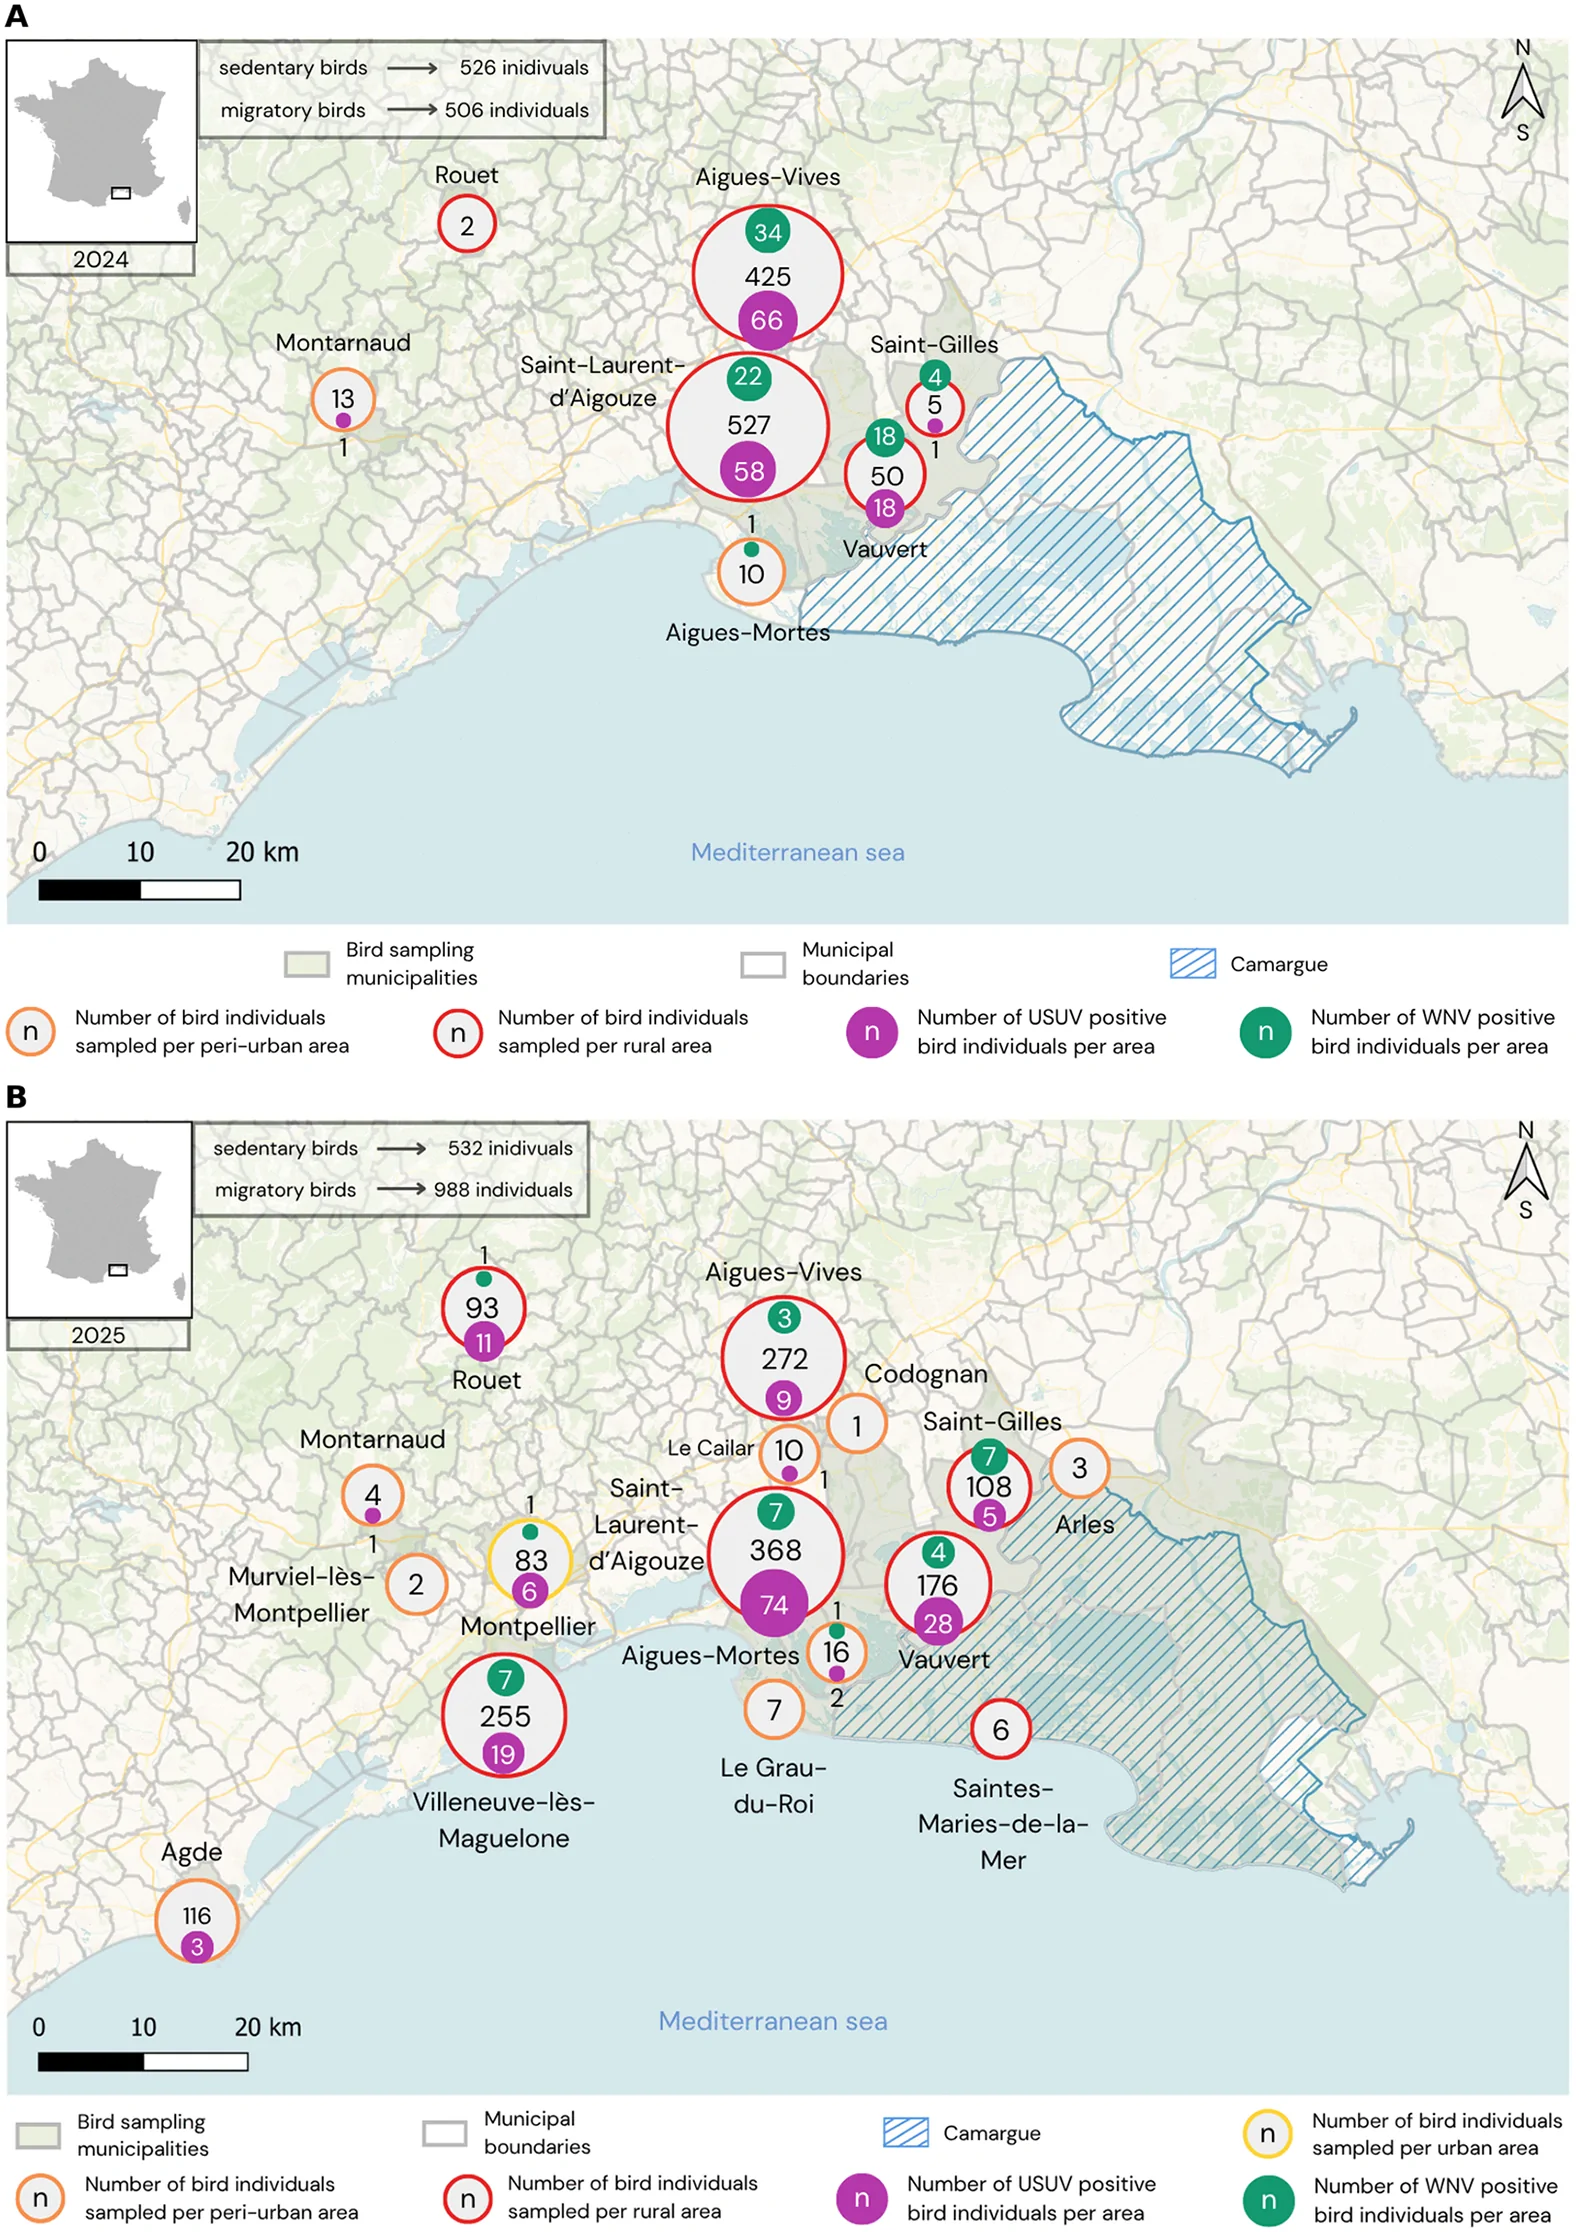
Spatial distribution of USUV and WNV infections in birds captured in 2024 (A) and 2025 (B). Each circle is centred on a sampling municipality; its size and inset number indicate the total number of individual birds captured there, and its outline colour indicates the locality type as defined in the Methods (yellow: urban; orange: peri-urban; red: rural). Smaller purple and green circles indicate, respectively, the number of USUV-positive and WNV-positive individuals identified at each site; their size is scaled to the number of positive individuals, so that positive counts can be interpreted relative to local sampling effort. Hatched areas delineate the Camargue wetland complex. A scale bar (0–20 km) and north arrow are provided. The inset map in the top-left corner situates the study area within France and summarises the total numbers of sedentary and migratory individuals sampled that year. Base map and overlay layers as described in Fig 1. Base map: CARTO Voyager No Labels (https://carto.com/basemaps/); map tiles CARTO, licensed CC BY 3.0 (https://creativecommons.org/licenses/by/3.0/); map data OpenStreetMap contributors, licensed under the Open Database License (ODbL) (https://www.openstreetmap.org/copyright; licence text: https://opendatacommons.org/licenses/odbl/1-0/index.html). Overlay layers: French municipal boundaries and Parcs Naturels Régionaux (PNR) boundaries, derived from OpenStreetMap and distributed via data.gouv.fr under ODbL. https://www.data.gouv.fr/datasets/parcs-naturels-regionaux-pnr-france-metropolitaine; https://www.data.gouv.fr/datasets/decoupage-administratif-communal-francais-issu-d-openstreetmap.
